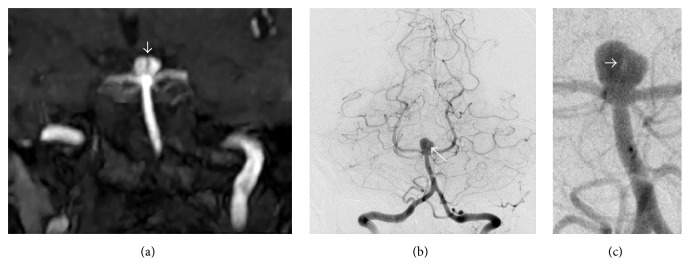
MRI demonstrating a saccular basilar tip aneurysm with a likely internal septum (a) which was confirmed on angiography (b) and its magnified view (c).
